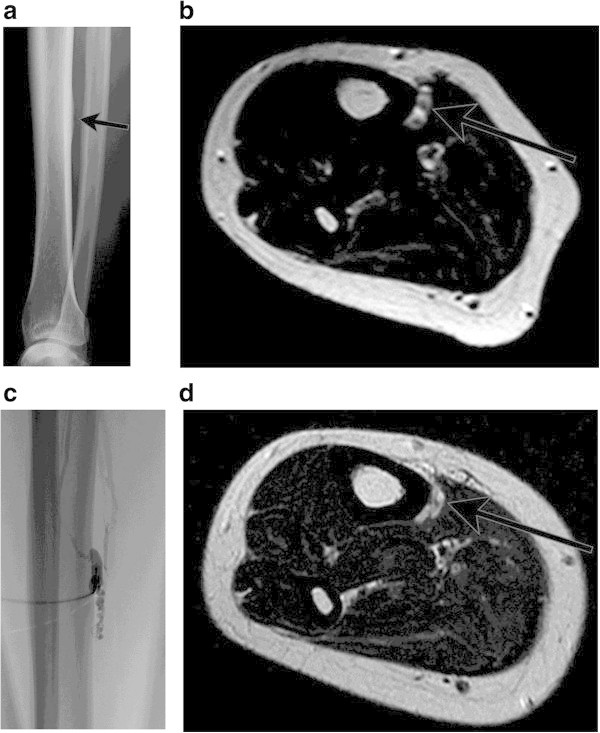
A 37-year-old-female with symptoms of severe pain of the right lower leg. A. Radiograph of the right lower leg demonstrates tiny periosteal reaction in the tibia (arrows). B. The axial T2-weighted MR image before treatment shows a tiny lesion adjacent to the tibia with cortical irregularity (arrows). C. Direct puncture phlebography reveals VM with normal veins and early venous return (Type 2). Sclerotherapy was performed with 3% polidocanol foam. D. Although the lesion decreased in size and signal intensity on MRI (arrows), she answered no change in symptoms and dissatisfaction on the questionnaire.
